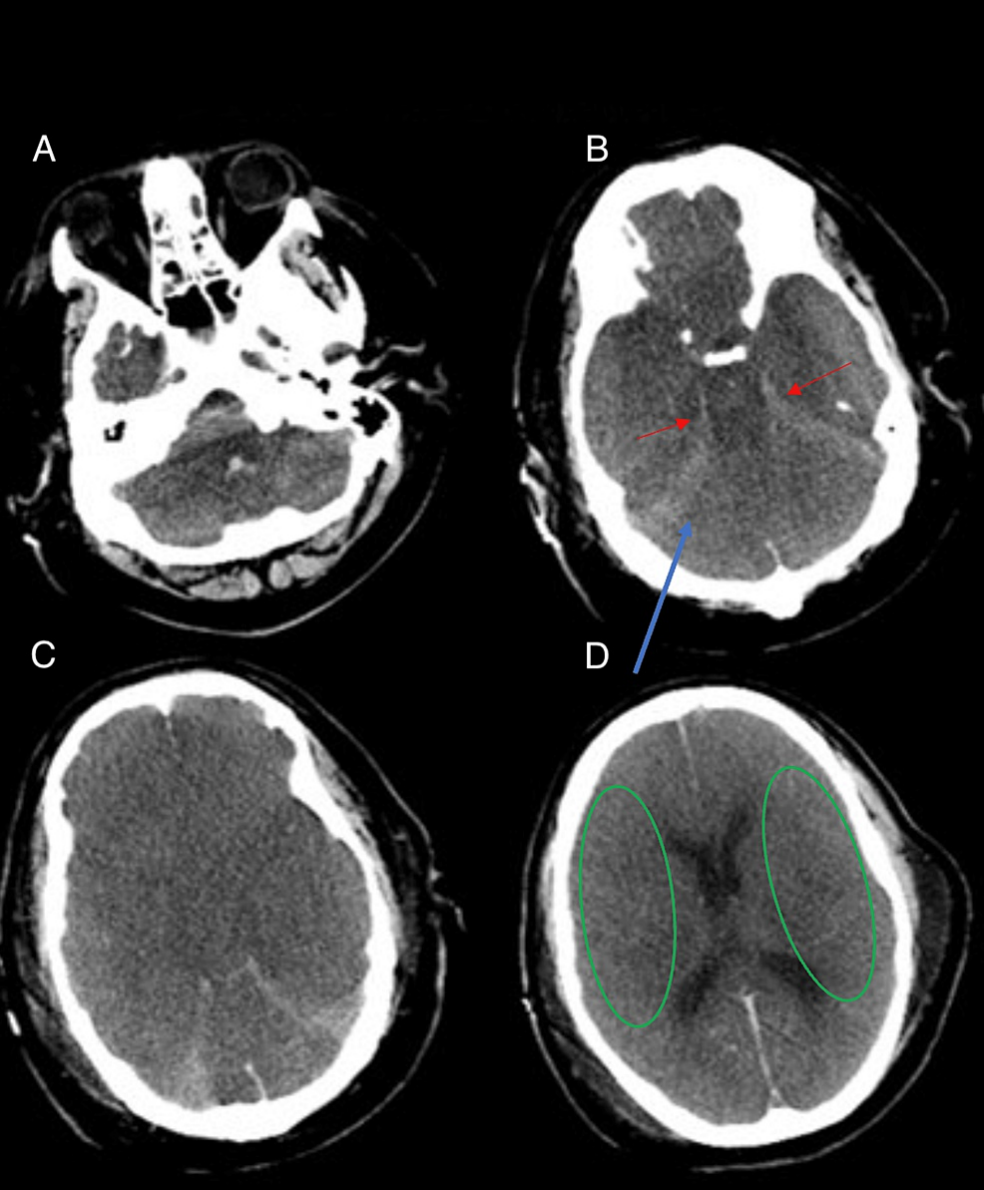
Four axial head images from a non-contrast head CT in a patient with diffuse anoxic ischemic injury. Panels A and C represent prior images taken for comparison. Panel B demonstrates a hyperdense area in the basilar cisterns (red arrows) and cerebellum (blue arrow). Panel D shows surrounding areas of diffuse sulcal effacement secondary to the anoxia (green circles).
